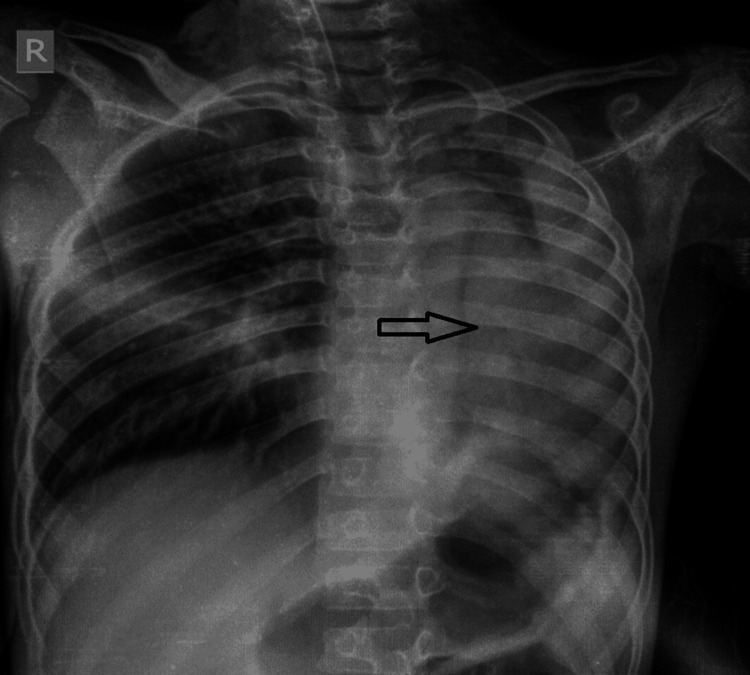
Chest X-ray showing a mediastinal shift toward the left side (black arrow) with volume loss due to left lung collapse
